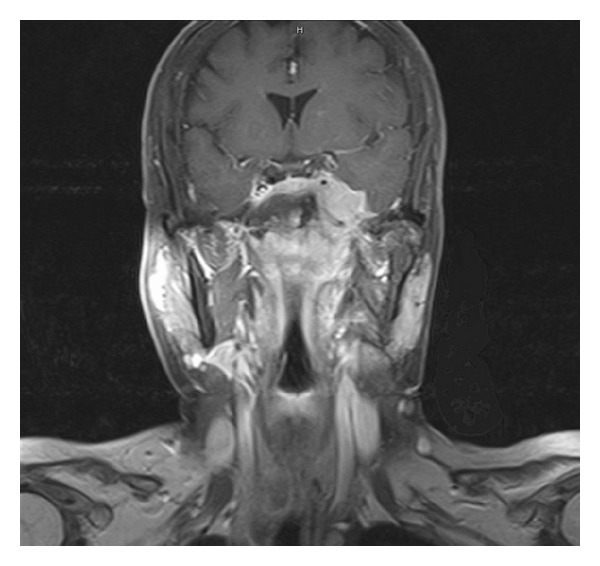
A representative case of ascending-type NPC. Coronal spin echo-enhanced T1-weighted MR image shows nasopharyngeal tumor with upward invasion into left cavernous sinus and medial temporal lobe. Small subclinical lymph nodes are noted at left neck.
